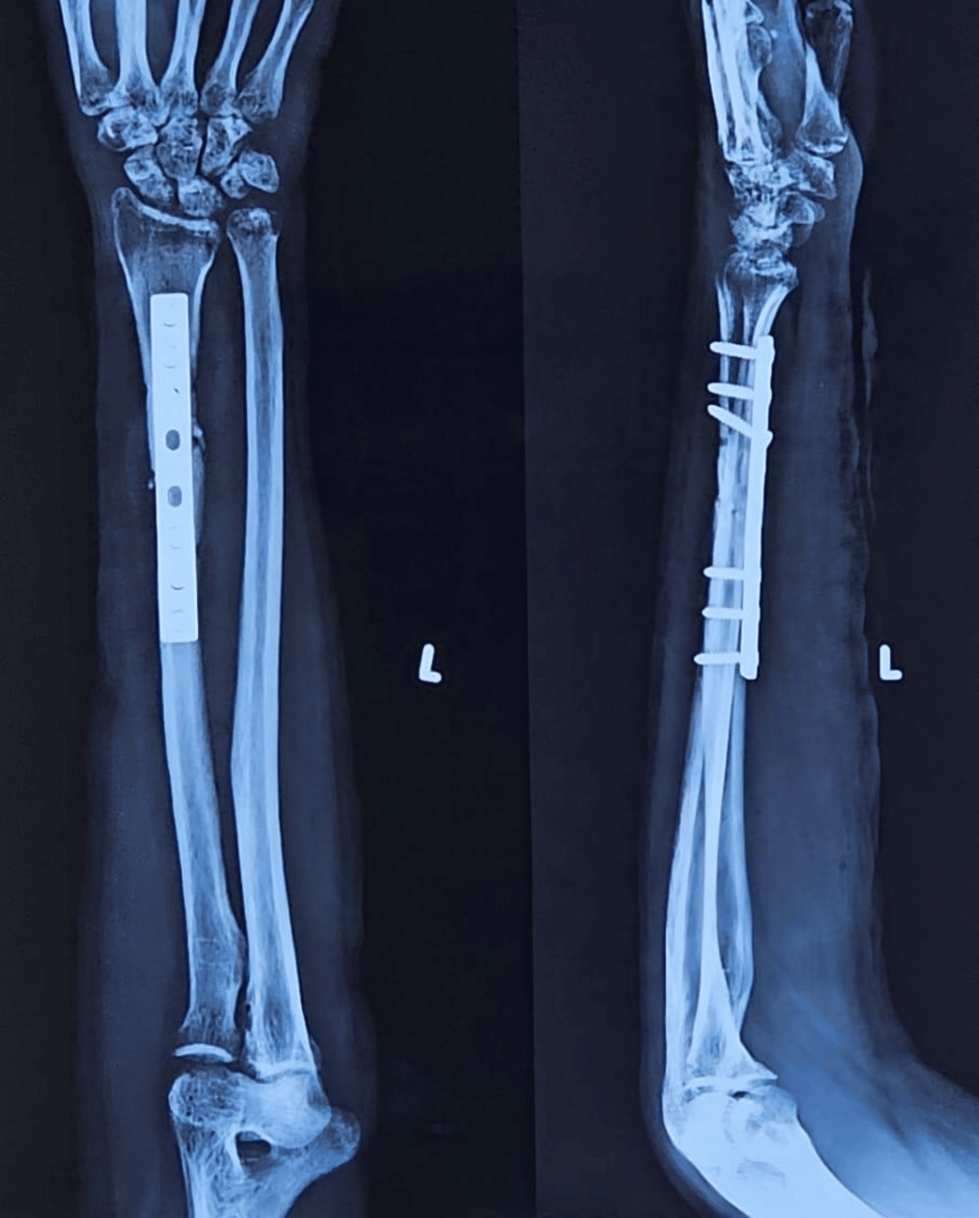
Postoperative X-ray of fracture radial shaft managed with dynamic compression plate.
